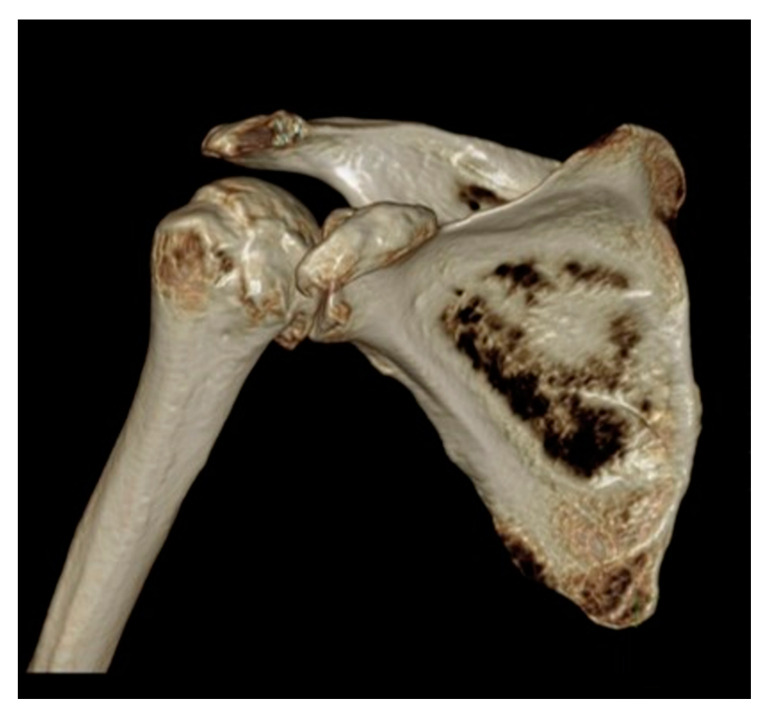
Three-dimensional reconstructed CT image of the shoulder in a patient with glenohumeral osteoarthritis, for preoperative planning.
